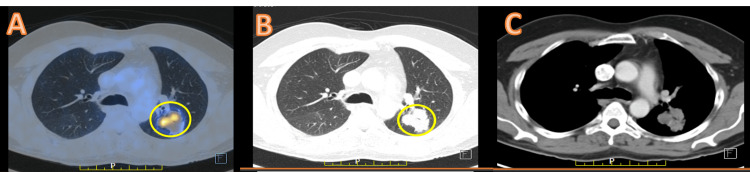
(A-C) Positron emission tomography-computed tomography scan suggestive of local disease progression with an increase in the size and metabolic activity of the left lung in the upper lobe.
